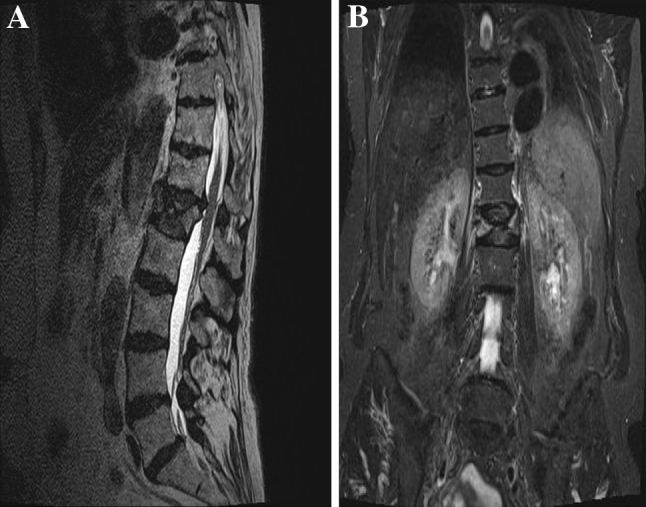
MRI showing the damaged both endplates with the retropulsed fragment
